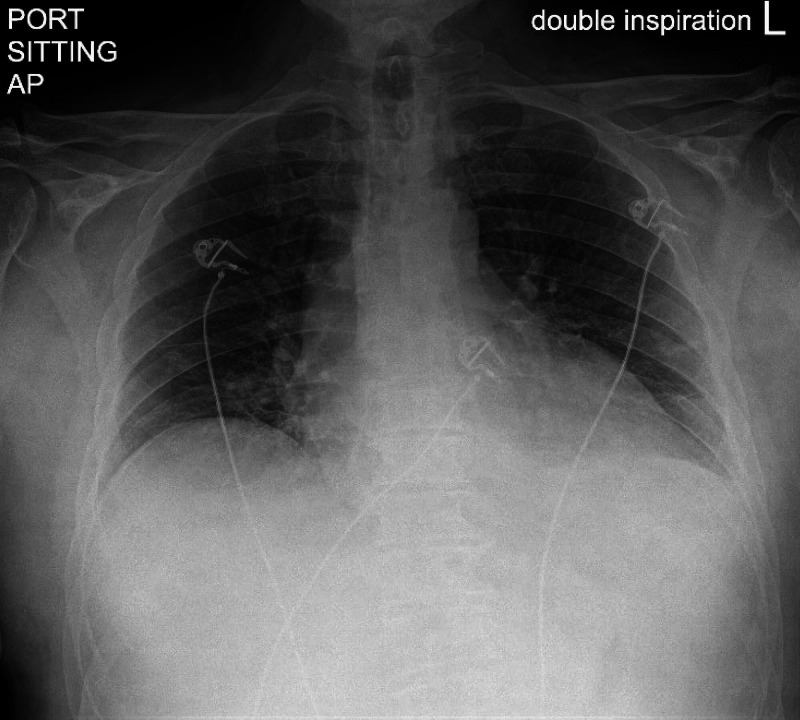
Chest radiograph showing enlarged cardiac silhouette and bibasilar atelectasis.
